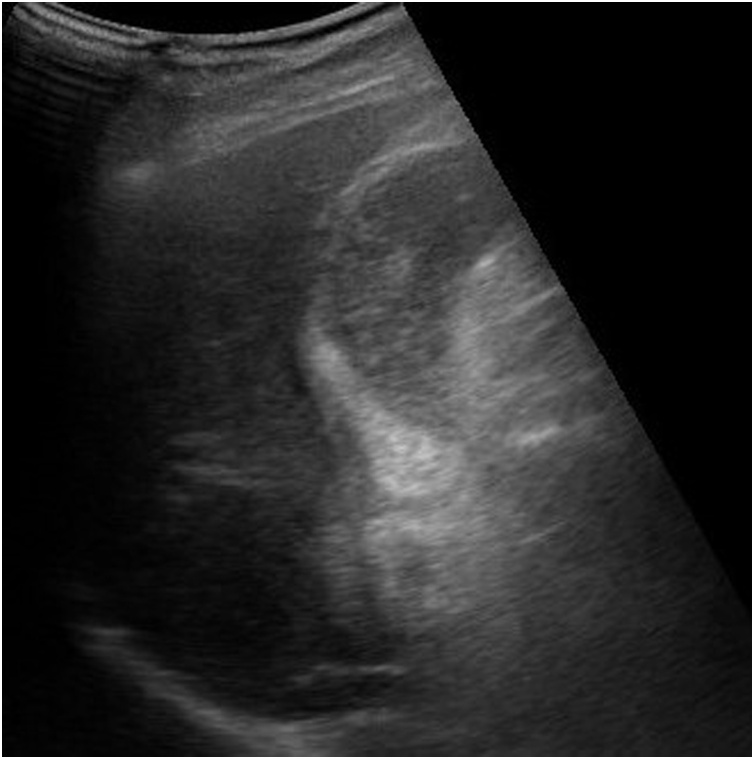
Ultrasonographic findings. Abdominal ultrasonography showed thickening of the gallbladder wall. Gallstones were not observed in the gallbladder.
